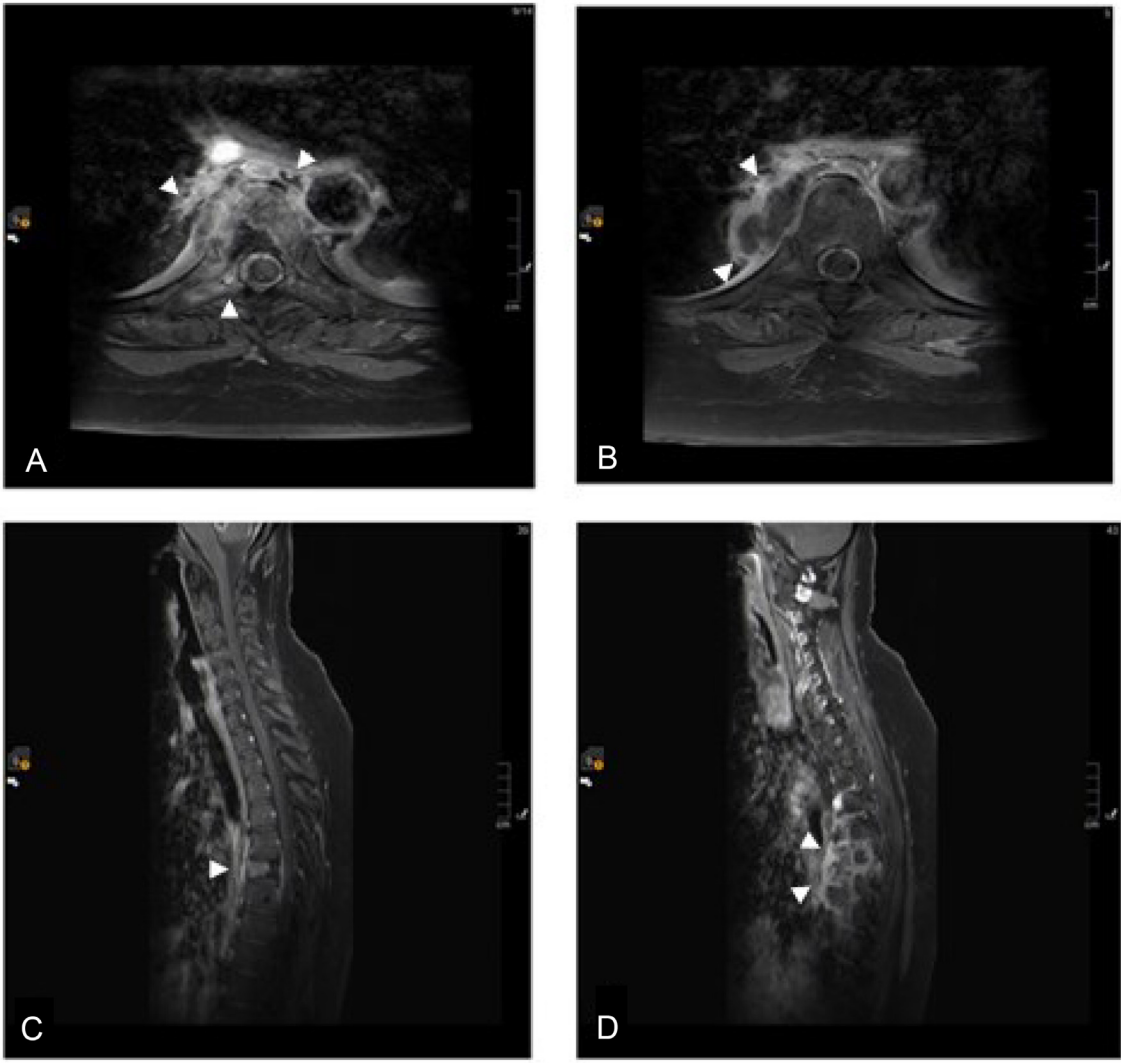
Axial (A and B) and sagittal (C and D) post gadolinium MRI of the thoracic spine showing infiltrative osseous lesion involving T6 vertebral body, extending to the right pedicle and transverse process sparing the adjacent disks, with right paravertebral peripherally enhancing collection extending from T5-T7 levels (arrow heads).
